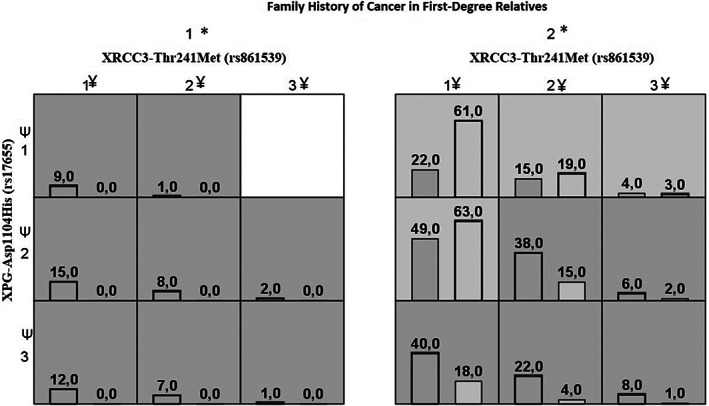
XRCC3‐Thr241Met (rs861539), XPG‐Asp1104His (rs17655) and family history of cancer in first‐degree relatives combined are associated with high and low risks of breast cancer multifactor dimensionality reduction (MDR) analysis with the highest testing accuracy. For each multifactor cell, the number of breast cancer cases is displayed in the left bar and the number of controls is displayed in the right bar. Cells of dark gray indicated high risk combinations and cells of light gray indicated low risk combinations. Family history of cancer in first‐degree relatives: 1* positive, 2* negative; XRCC3‐Thr241Met (rs861539): 1^¥^ Thr/Thr (CC) genotype, 2^¥^ Thr/Met (CT) genotype, 3^¥^ Met/Met (TT) genotype; XPG‐Asp1104His (rs17655): 1^Ψ^ Asp/Asp (GG) genotype, 2^Ψ^ Asp/His (GC) genotype, 3^Ψ^ His/His (CC) genotype
